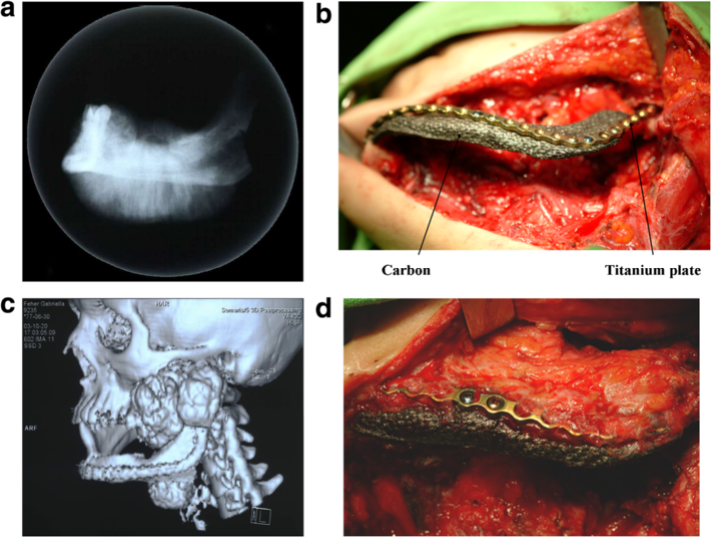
The Carbulat™ plate had to be removed because of tumour recurrence. a X-ray picture of a huge osteosarcoma of the mandible. Very characteristic sclerotizing form. b Carbulat™ and titanium plate reconstruction. c 8 months after the first surgery the relapsed tumour (CT picture). d After removal the relapsed tumour the Carbulat plate. It is visible that the Carbulat™ plate integrated extremely well into the tissues
